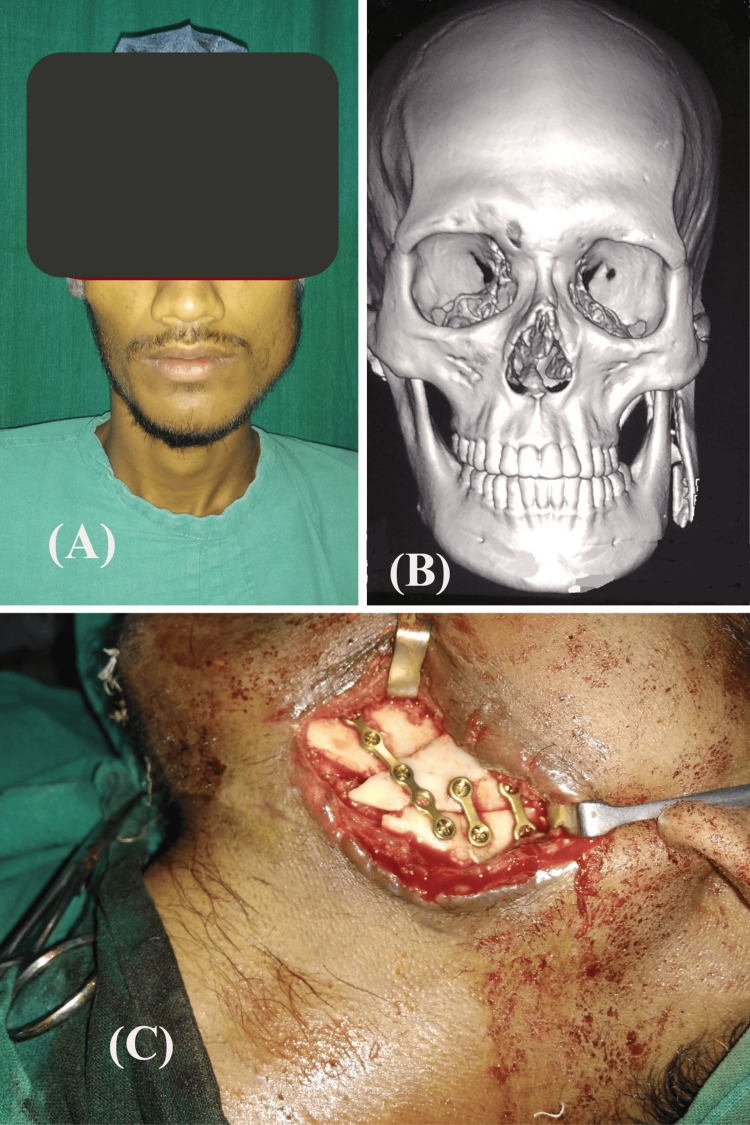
Fracture angle of the mandible: (A) preoperative picture of the patient; (B) CT scan showing the fracture; and (C) fracture stabilization with mini plates.
